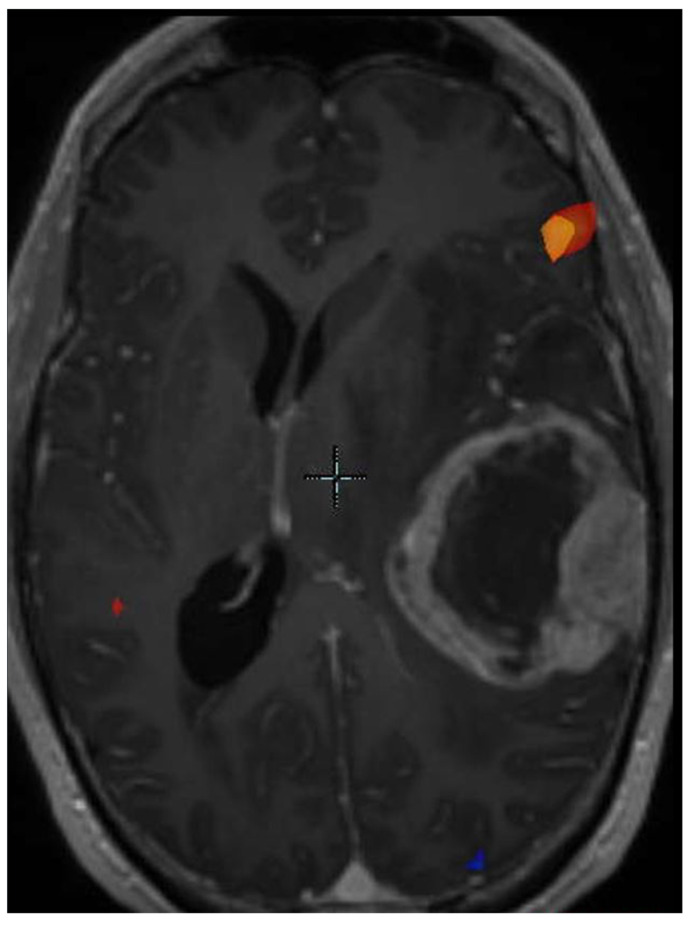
Functional MRI BOLD map superimposed on an MRI of an epithelioid GBM. Note the large cystic component.
